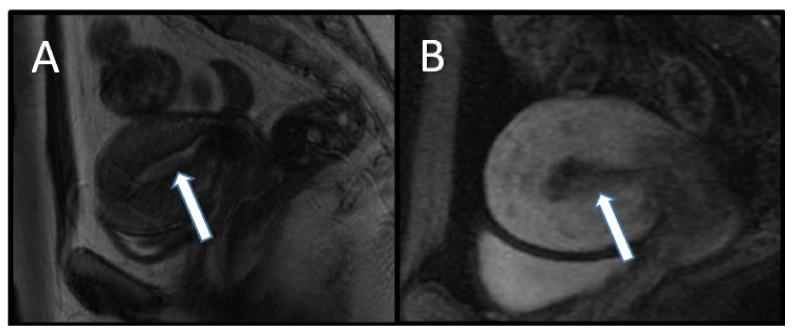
An 18-year-old woman with Cowden syndrome who underwent surveillance biopsy for dysmenorrhea and irregular menstrual bleeding. Biopsy-proven grade 1 endometrioid endometrial carcinoma was found, and staging magnetic resonance imaging (MRI) was performed. (A) Sagittal T2-weighted and (B) contrast-enhanced T1-weighted MR images show endometrial thickening (arrows) measuring approximately 2 cm. The patient subsequently underwent hysterectomy, and the pathologic diagnosis was upgraded to grade 2 endometrioid endometrial cancer.
